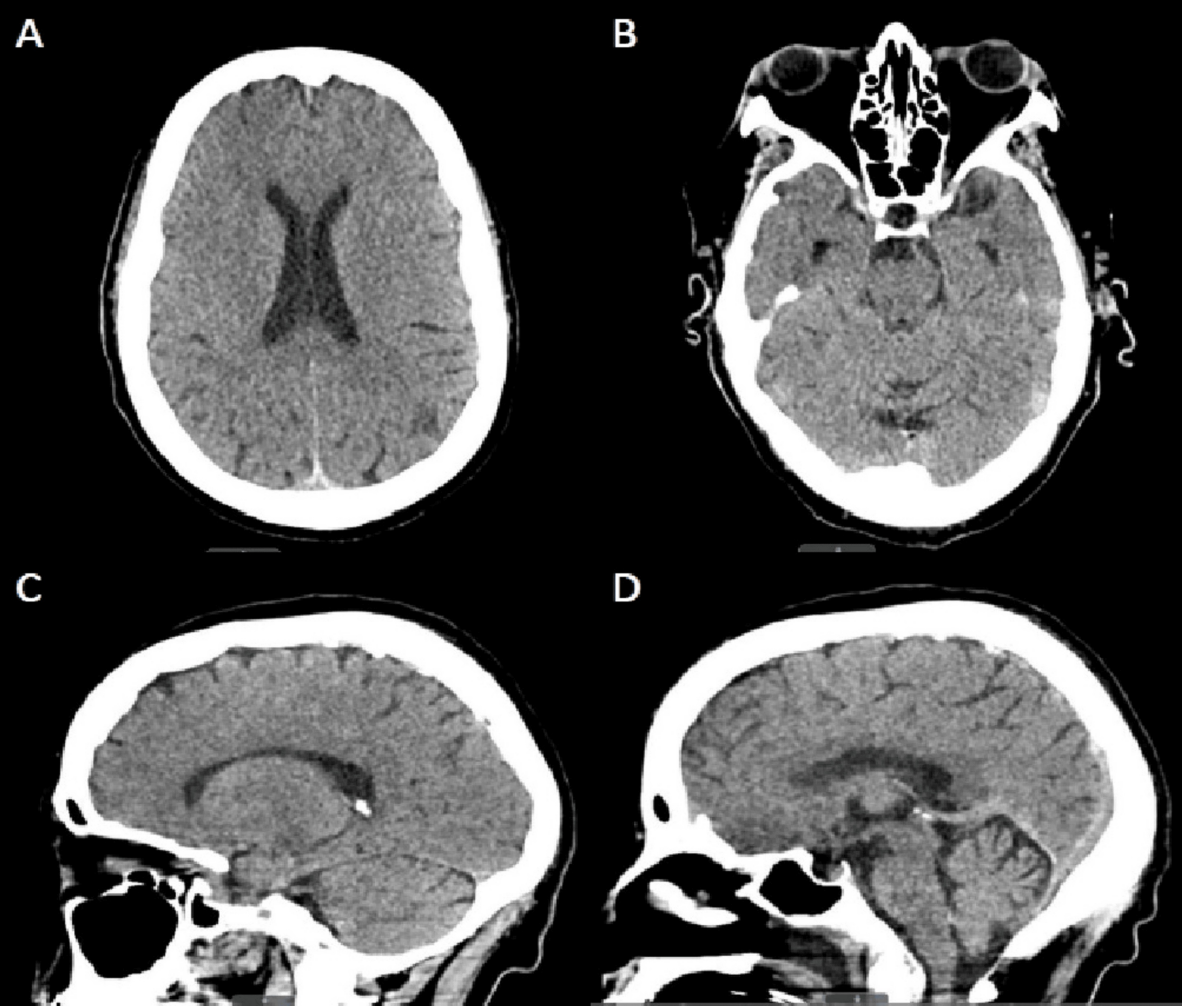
Non-contrast CT of the head showing no acute intracranial process. (A) Axial image at the level of the lateral ventricles; (B) Axial image at the level of the pons; (C) Sagittal image at the level of the thalamus; (D) Sagittal image at the level of the central sulcus.
